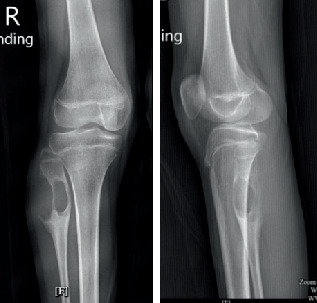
Biplanar plain radiographs of the right knee.
